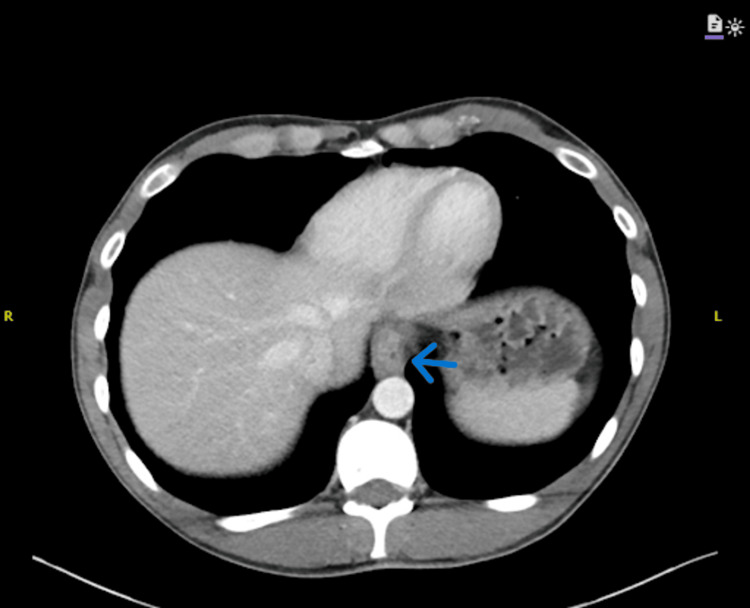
Non-contrast CT demonstrates thickening of the distal esophagus (arrow).
